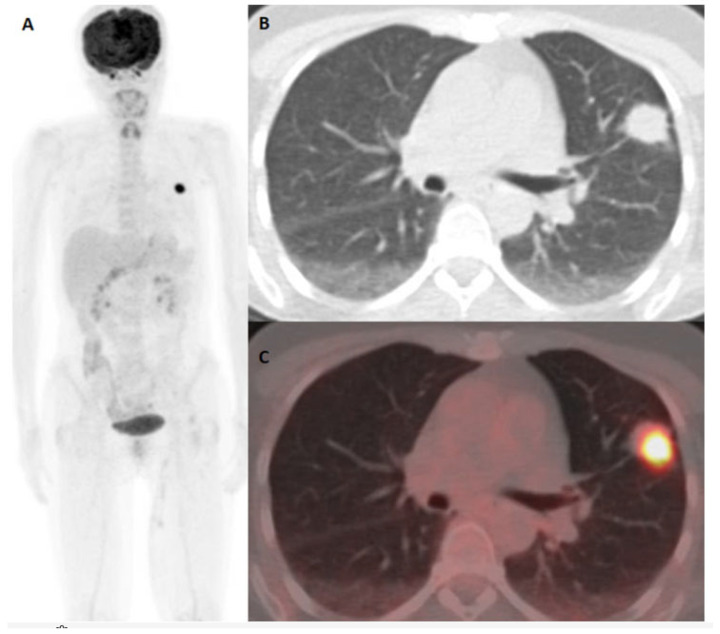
A 55-year-old female with cutaneous melanoma of the left thigh (Breslow 3.7 mm, pT3b, stage II). 18F-FDG PET/CT performed for staging purposes. Nodular lesion in the left lung proved to be an additional synchronous lung cancer. MIP 18F-FDG PET/CT shows a nodular lesion in the left lung (A). Axial chest CT (B) and fused PET/CT on the axial plane (C) demonstrate an 18F-FDG avid lesion in the left lung.
